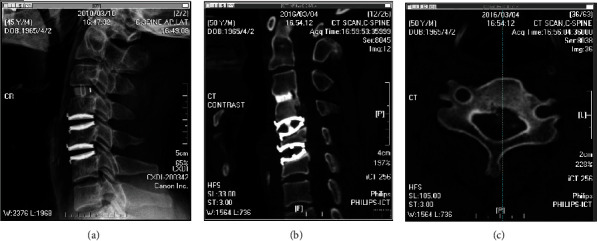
Sample patient showing osteolysis. (a) Lateral radiograph of a 45-year-old man taken two months after cervical disc arthroplasty with Bryan Discs over the C4-C5 and C5-C6 levels. (b, c) Computed tomography scan of the same patient 5 years postoperation found osteolysis over the posterior aspect of the C4 body.
